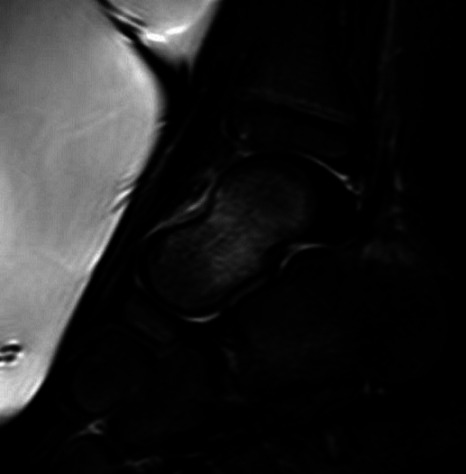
A T2 weighted MRI of the talus. 1) loss of cortical continuity and 2) increased signal in the talus indicating marrow oedema.
